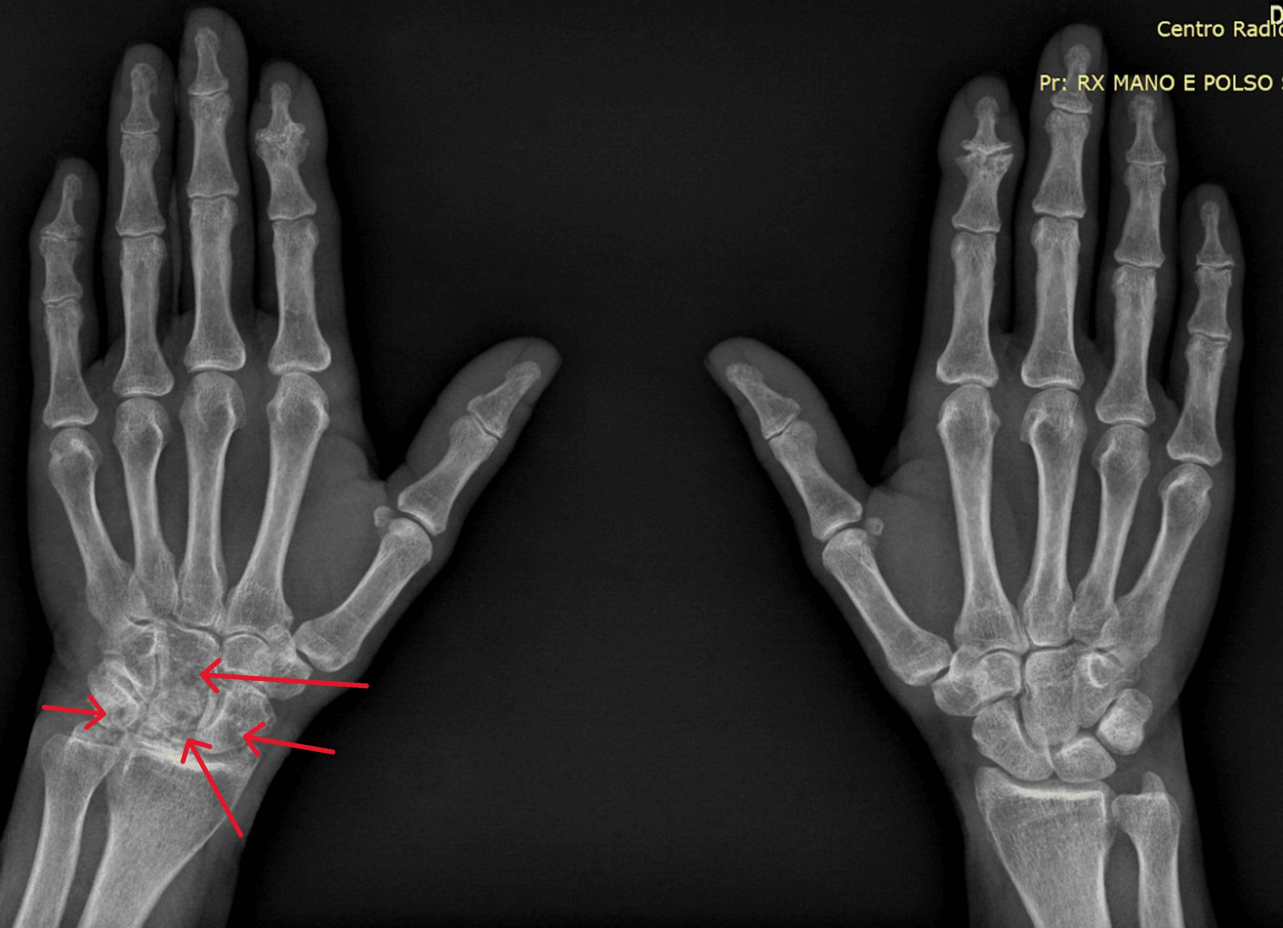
Follow-up X-ray of the left hand after one year, showing progression of radiographic damage in the left wrist with new erosions and geode formations
